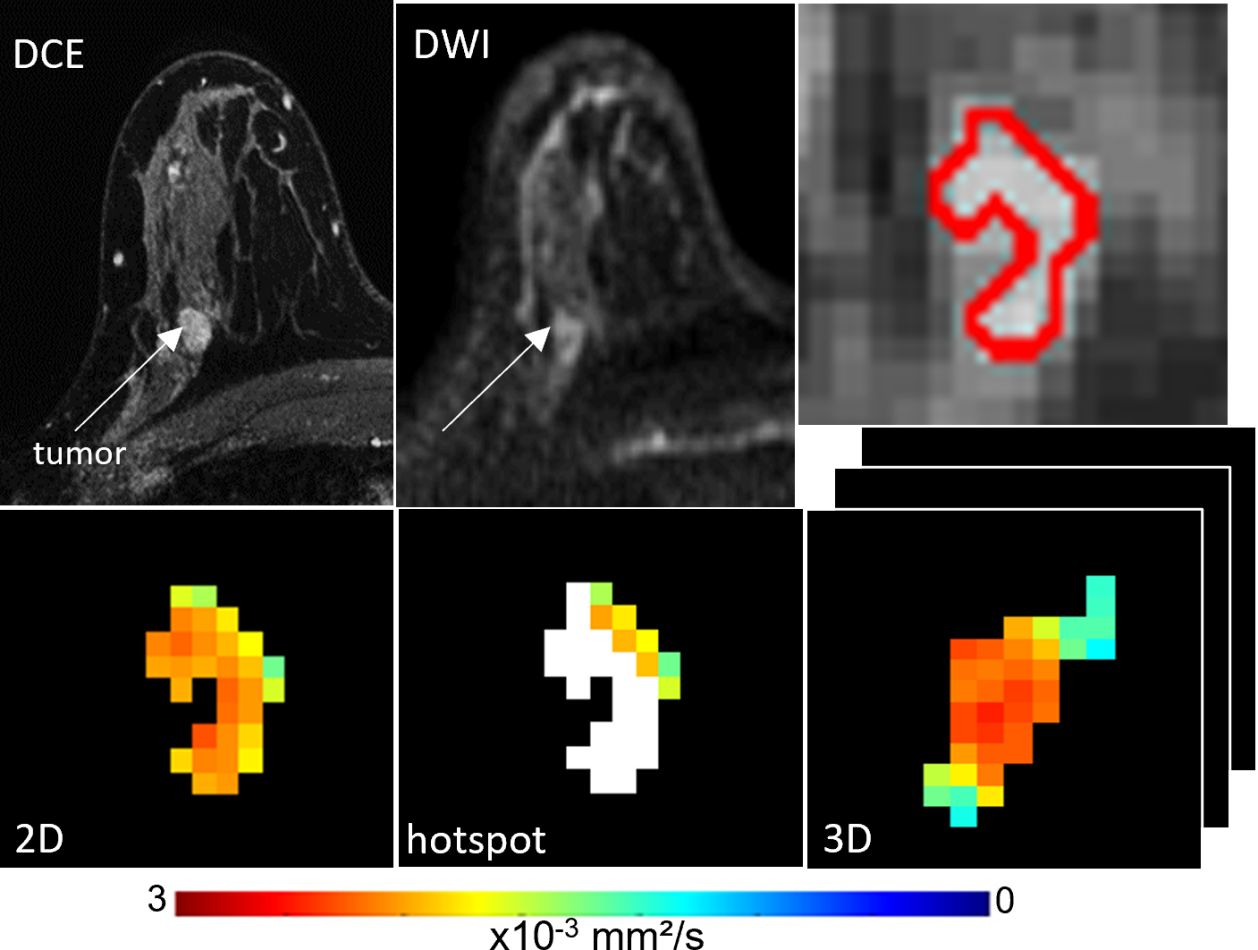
ADC measures of a BI-RADS 4 11 mm mass detected in a 41-year-old woman who underwent screening MRI. Lesion ADCs calculated using the different ROI techniques were 2.12, 1.84, and 1.97 x10^-3^ mm^2^/s for 2D, hotspot, and 3D ROIs, respectively. On biopsy, it was found that the lesion was benign breast tissue with focal fibrocystic changes.
